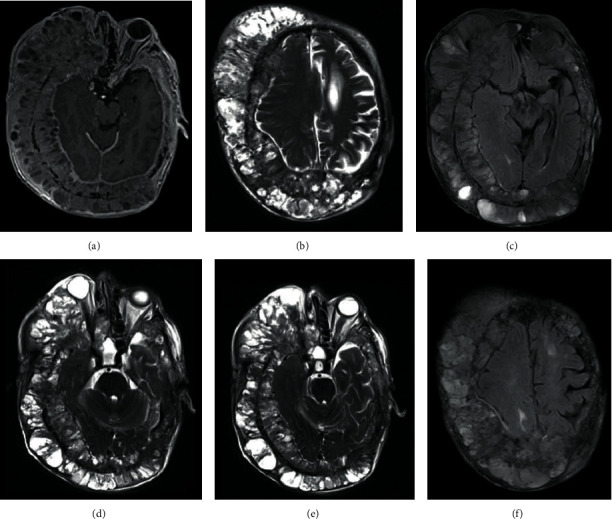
3.0 T MRI with and without administration of paramagnetic contrast agent. On axial postgadolinium T1w-FFE image, there is evidence of (a) an extensive enhancing lesion of the scalp showing, in axial T2-TSE images, infiltration of (b) skin, bones, dura mater, and subdural spaces at the level of the right middle and inferior frontal gyrus. Compression of the right orbital and straight gyrus is also noted, as seen on axial FLAIR-T2w images (c). Axial T2-TSE images show also that (d) the right orbit is fully invaded by the lesion, with proptosis of the globe, which appears compressed, and compression of the optic nerve and that (e) there is invasion of ethmoidal cells, pterygopalatine fossa, and foramen rotundum. Axial FLAIR-T2w images show elevated signal intensity in some white matter fibers, like the right frontal lobe (f).
